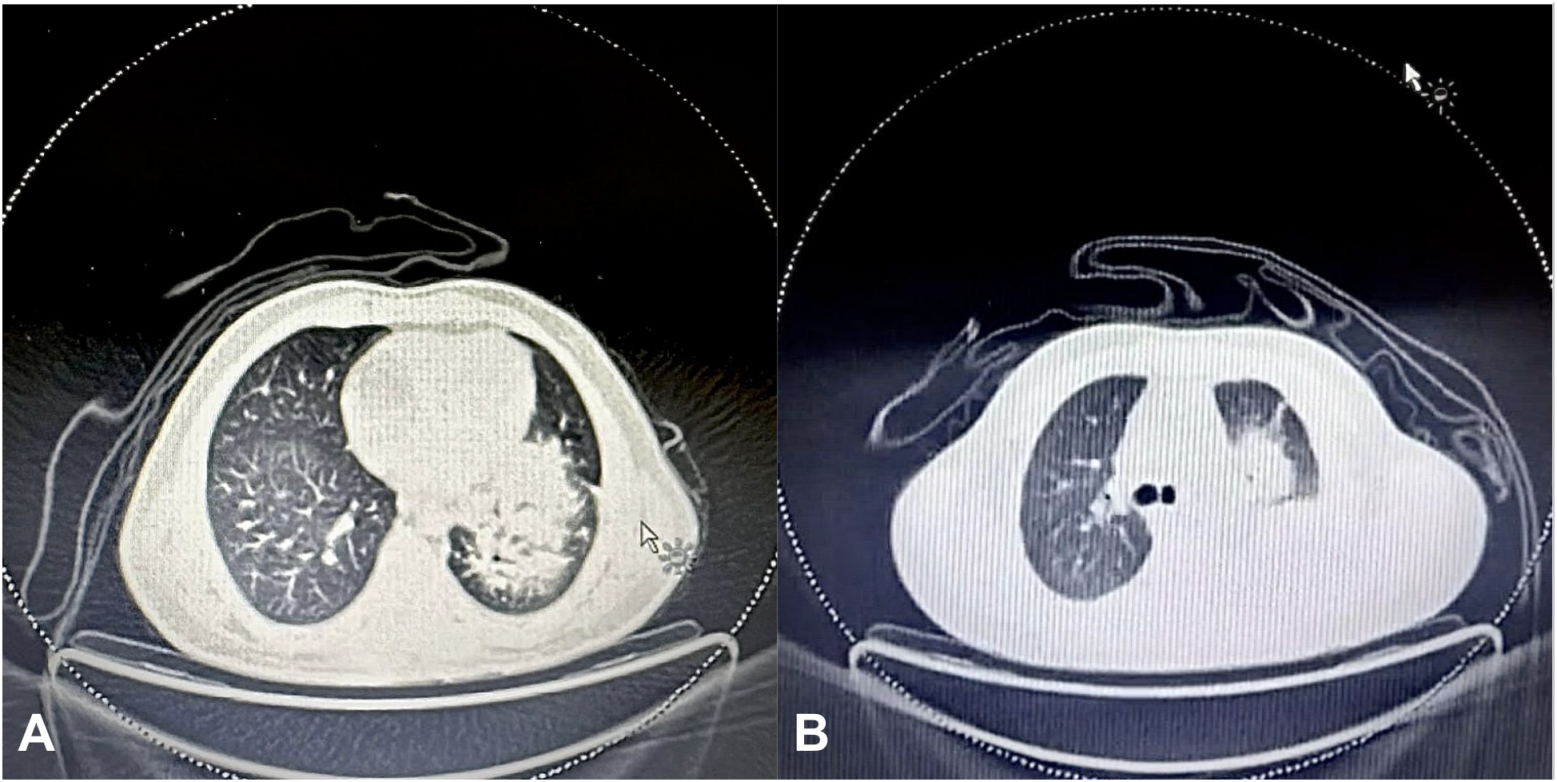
(A) Initial FDG PET scan of the chest showing an 11x11x10 cm soft tissue mass in the left lung perihilar region, encasing the left lobar bronchus and extending from the medial pleural surface to the lung periphery. (B) Final FDG PET scan showing a marked interval increase in the size of the soft tissue mass in the left lung perihilar region (12x12x8 cm), extending into the upper and lower lobes and reaching the pleural and pericardial surfaces.
